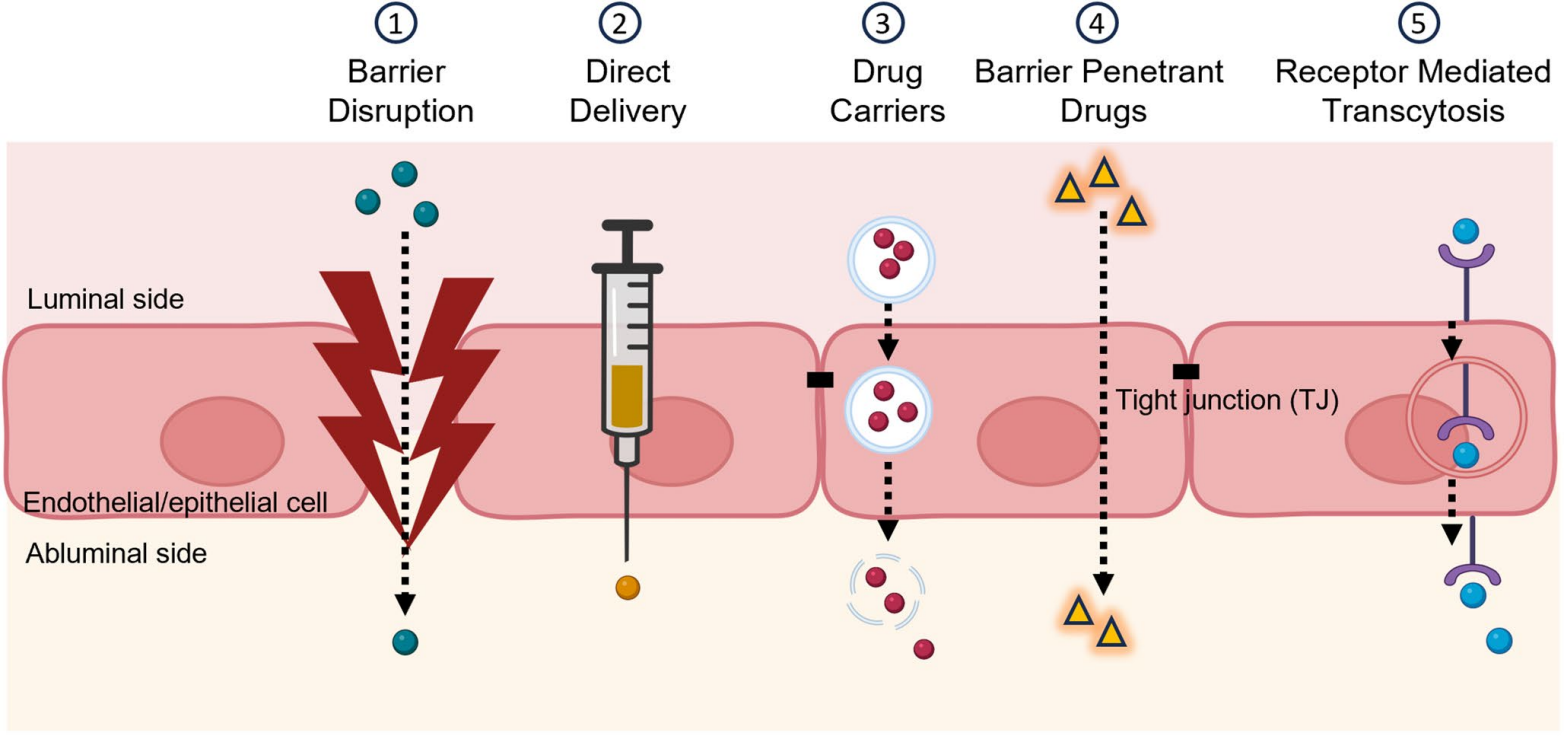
Routes of drug delivery across the blood-brain barrier (BBB) or blood-retinal barrier (BRB). Several approaches exist to deliver drugs across the BBB or BRB. This includes disruption of the barrier via localised ultrasound (1); direct delivery into the eye (intravitreal injection) or brain (intrathecal or intracerebral injection) (2); packaging drugs into vesicles to help cross the barrier (3); developing small lipophilic molecule that can enter the brain freely (4) or through receptor mediated transcytosis (5). Figure modified from Zelek and Morgan 2022.
